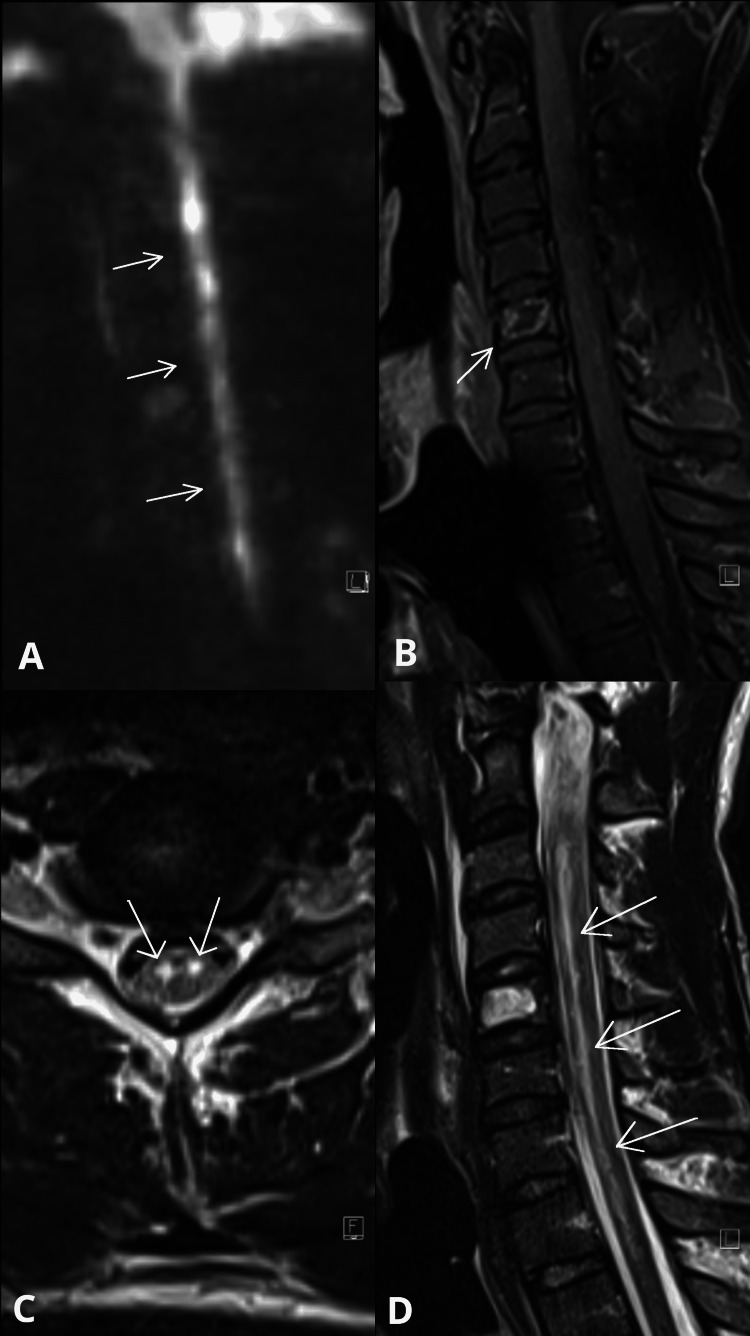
MRI of the cervical spine demonstrating intramedullary spinal cord lesions (A): Sagittal diffusion-weighted imaging (DWI) sequence showing a hyperintense linear lesion extending from C2 to D1, consistent with acute infarction in the anterior spinal artery territory. (B): Sagittal gadolinium-enhanced T1 image demonstrating C5 vertebral body lesion with peripheral enhancement after gadolinium administration. (C): Axial T2 section showing central hyperintensity involving the anterior two-thirds of the cord, with relative sparing of the posterior columns, consistent with anterior spinal artery infarction. (D): Sagittal T2-weighted image highlighting the involvement of the mid-to-lower cervical cord with well-demarcated intramedullary hyperintensity.
